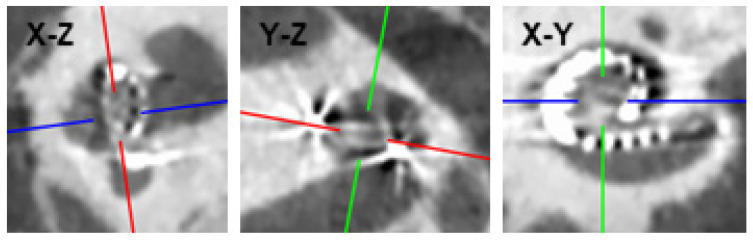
Example of a CT scan of a cochlea with an inserted MidScala electrode array (P1). The red lines in the *x-z* and *y-z* planes are aligned with the cochlea’s basal turn so that the *x-y* plane is aligned with the cochlea. One or more slices in the *x-y* plane, showing the CI electrodes’ contacts, can then be exported for analysis.
